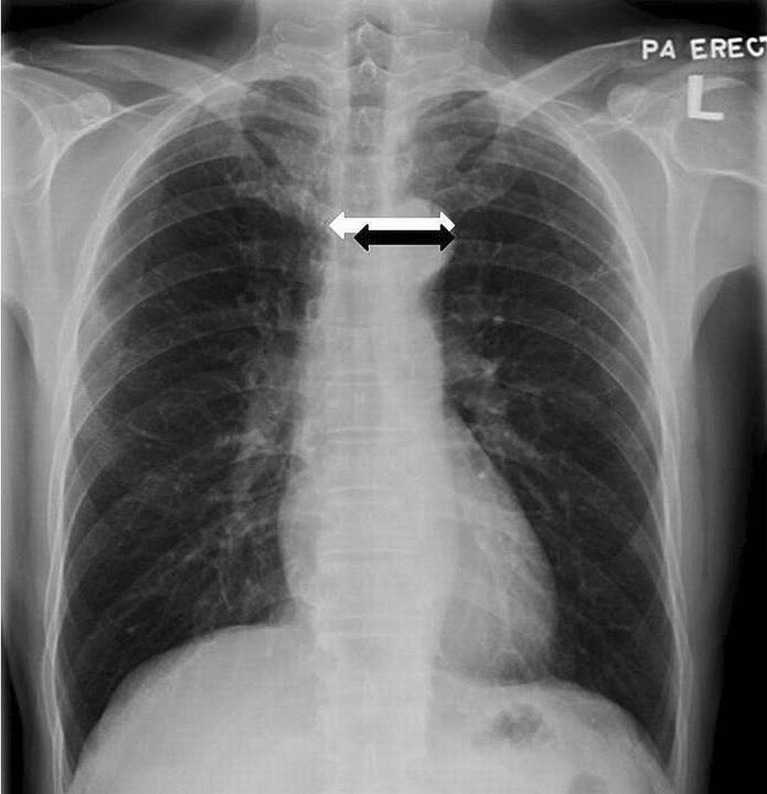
PA chest radiograph showing mediastinal measurement: MW (*white arrow*) and LMW (*black arrow*)
